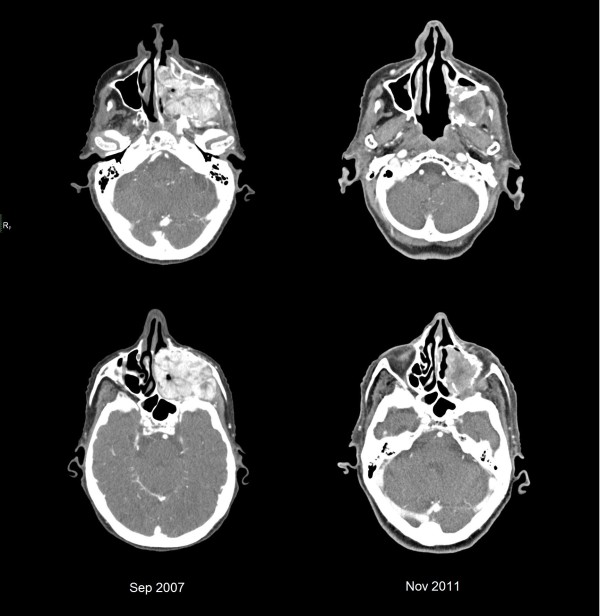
Sequential computed tomography between September 2007 and November 2011 showing decrease in tumour size after a short course of radiation therapy delivered in June 2009.
